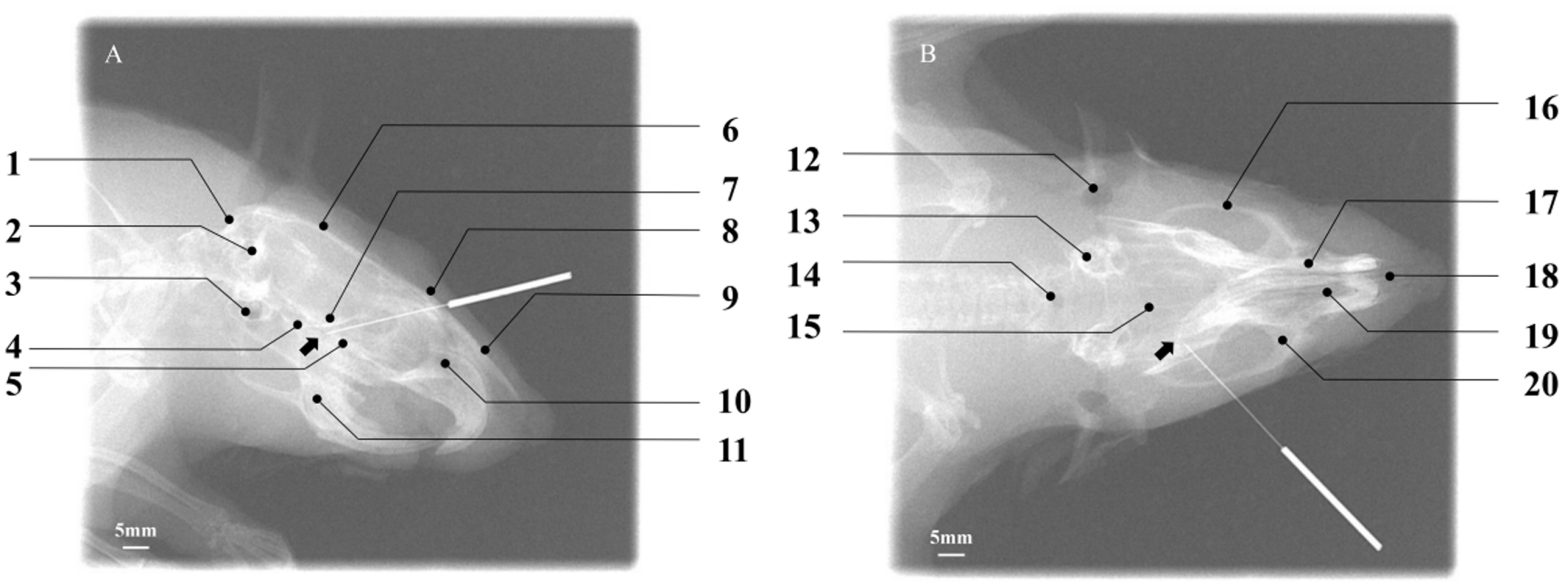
Verification of acupuncture needle placement within the pterygopalatine fossa using digital X-ray fluoroscopy. (A) Lateral view: the needle tip (NT) is positioned within the target PPF region, with the black arrow indicating the location of the sphenopalatine foramen. Key cranial landmarks are annotated: (1) occipital bone; (2) foramen magnum; (3) auditory structures/otic region; (4) basisphenoid bone; (5) palatine bone; (6) parietal bone; (7) presphenoid bone; (8) frontal bone; (9) nasal bone; (10) nasal cavity; (11) mandible. Scale bar: 5 mm. (B) Coronal view: the NT is located within the target PPF, with the black arrow indicating the sphenopalatine foramen. Annotated cranial landmarks include: (12) external auditory meatus; (13) internal ear; (14) foramen magnum; (15) parietal bone; (16) zygomatic bone; (17) nasal bone; (18) nasal septum; (19) nasal cavity; (20) maxilla. Scale bar: 5 mm. The successful placement, as defined by its spatial relationship to surrounding cranial landmarks, confirms the accuracy of the established acupuncture pathway.
